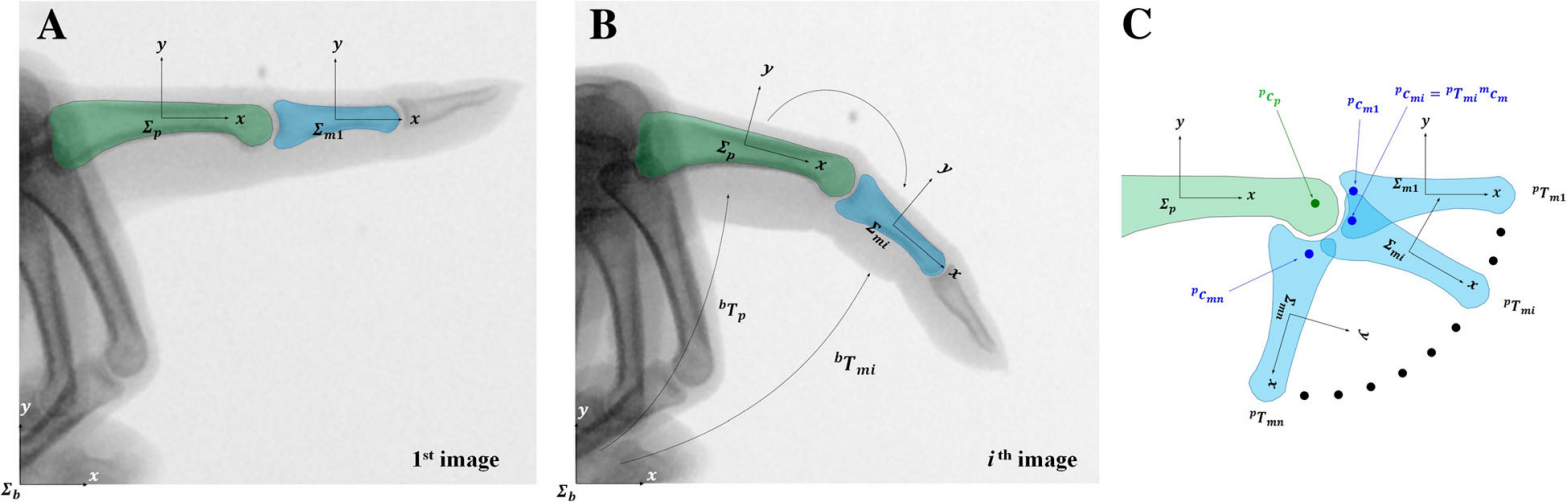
A three-dimensional model of the proximal interphalangeal joint (PIPJ) using the rolling contact joint mechanism. a Contours of the proximal (green) and middle (blue) phalangeal bones and the base (*Σ*_*b*_), proximal (*Σ*_*p*_), and middle (*Σ*_*m*1_) coordinate frames in full extension of the PIPJ. b The *i*th radiographic image and homogenous transformation (HT) matrices. c The average center of rotation (ACR) based on the HT matrices obtained from multiple radiographic images
